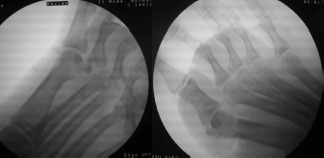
Intraoperative radiograph after reduction of first and then both MTP joint dislocation.
